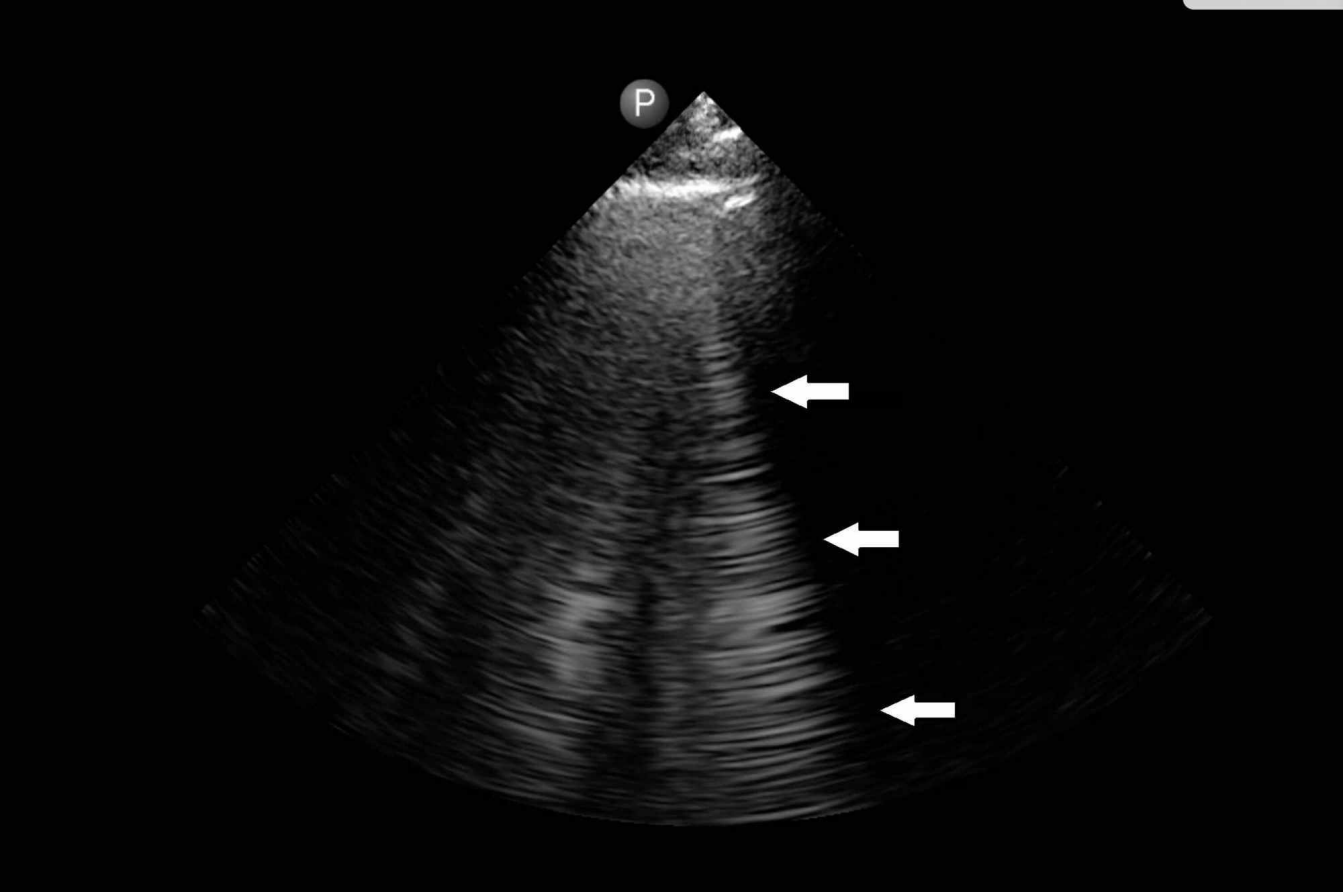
Lung Ultrasound, with multiple comet tails (also known as B-lines) One vertical comet tail (B-line) is indicated by the three white arrows.
